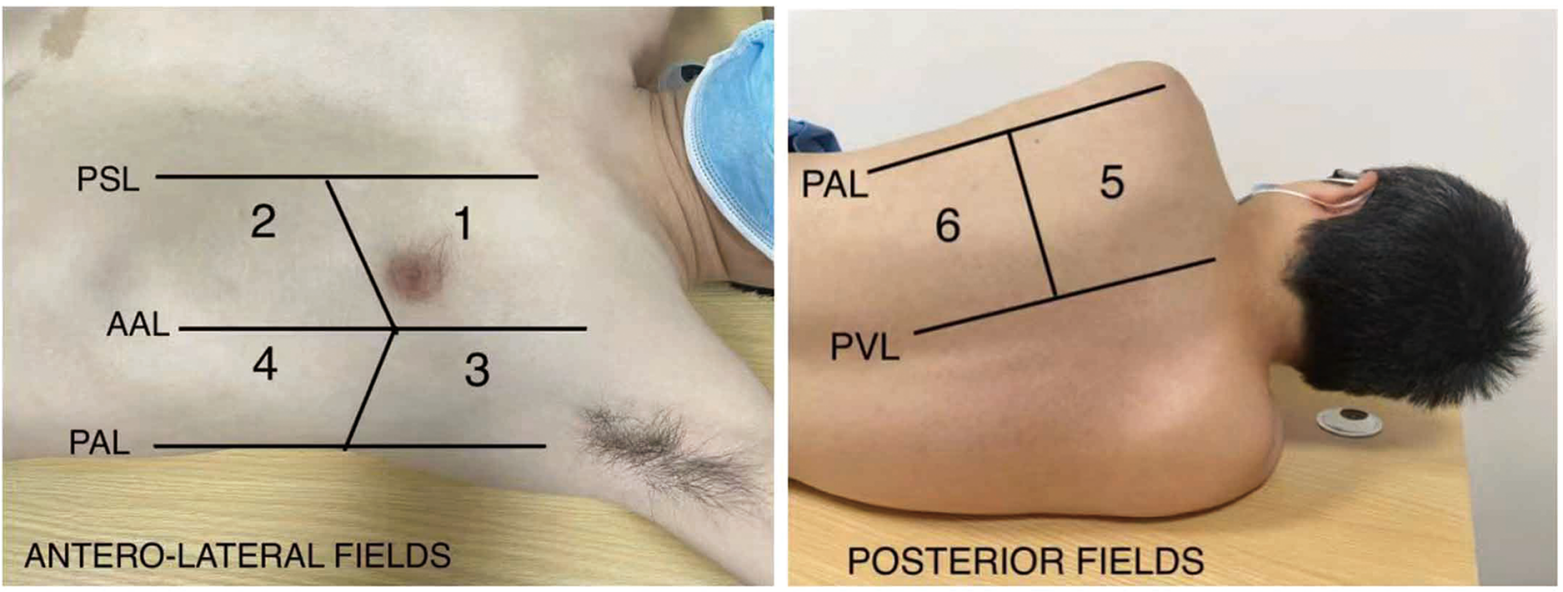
Lung ultrasound regions. PSL, parsternal line; AAL, anterior axillary line; PAL, posterior axillary line; PVL, paravertebral line.
